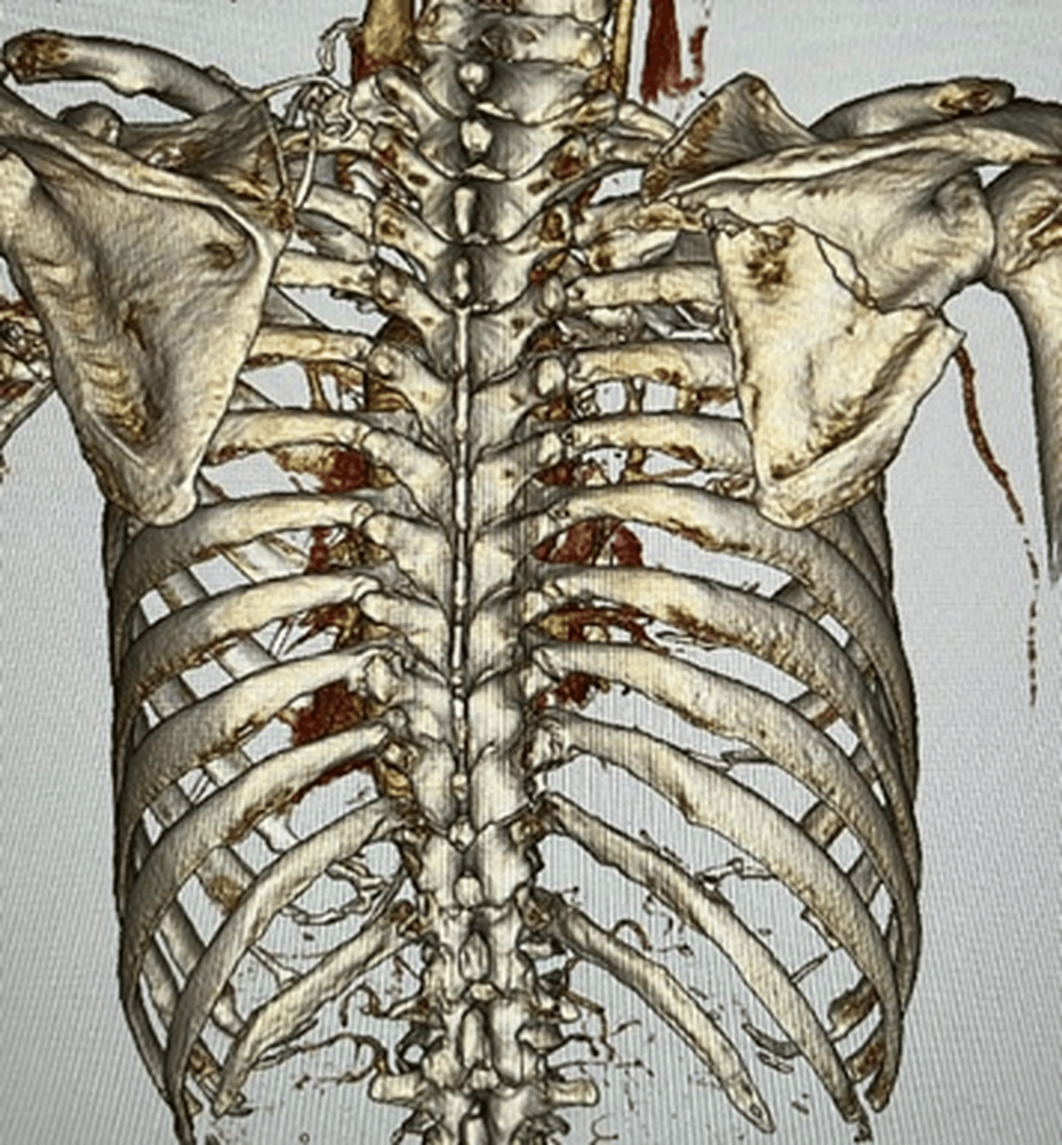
3D CT image on admission - 1 A fracture of the right scapular body was present, with multiple rib fractures accompanied by a flail segment just below it CT: computed tomography
